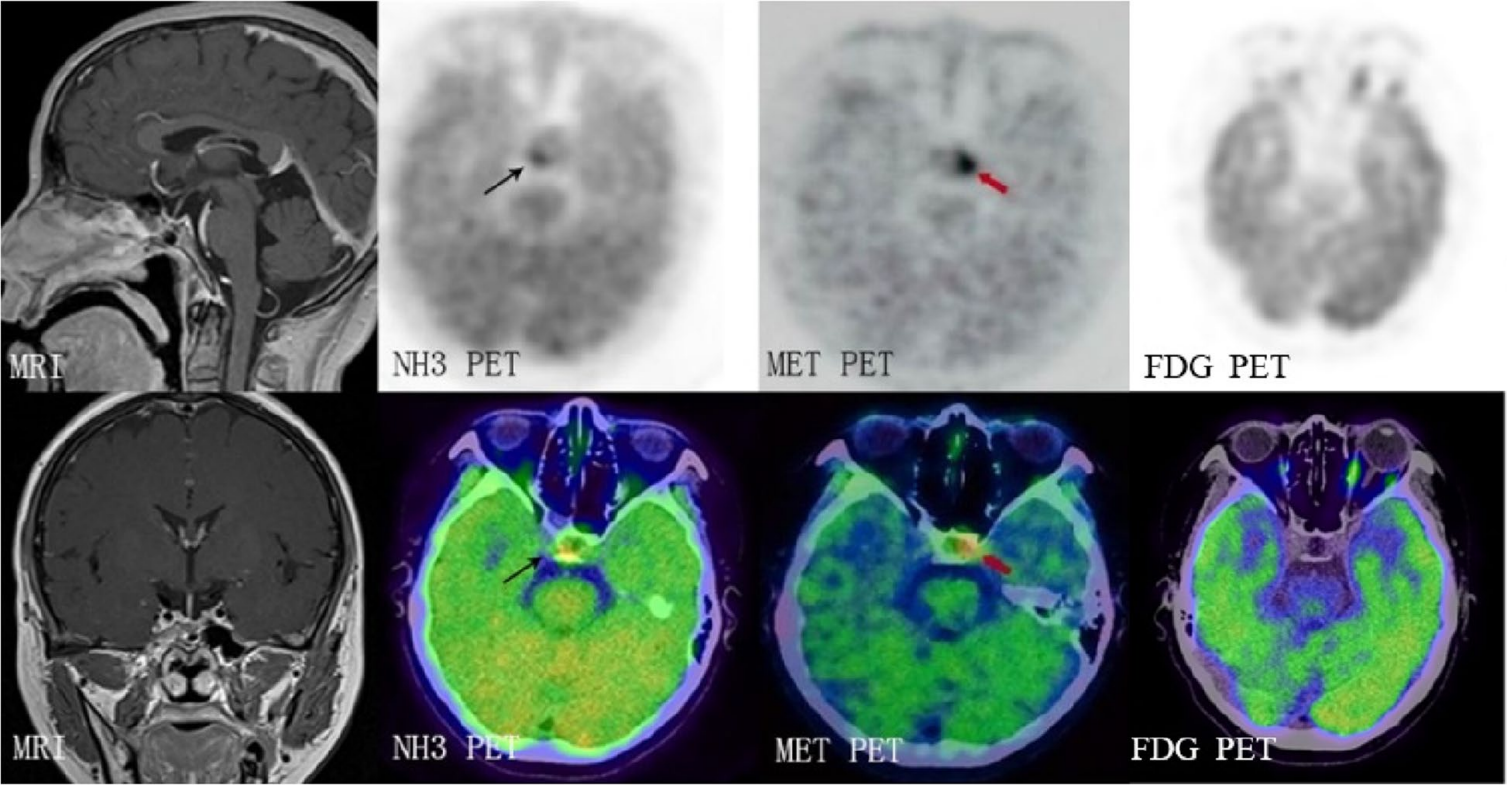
Comparison of [^11^C]C-MET to [^13^N]N-NH3 PET in the detection of residual PAs. Tumors were not detected by neither MRI nor [^18^F]F-FDG PET. [^11^C]C-MET and [^13^N]N-NH3 PET/CT images showed tumors (red arrow) and pituitary tissue (black arrow), completely differentiated by [^13^N]N-NH3 uptake and[^11^C]C-MET uptake
